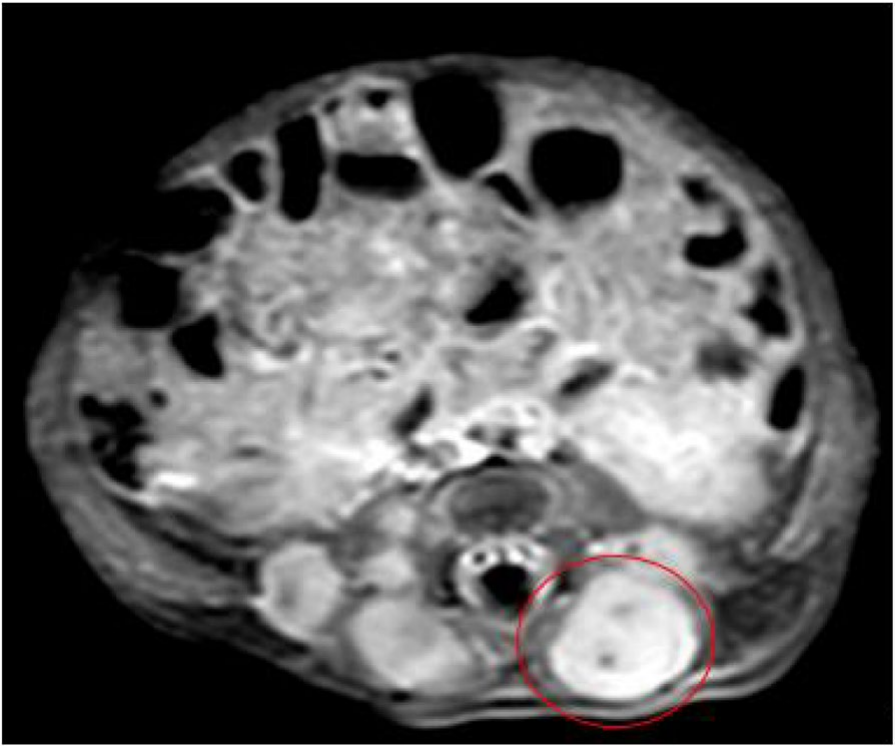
MRI at the age of 20 days: Right sacral lesion in axial T1 with contrast enhancement
